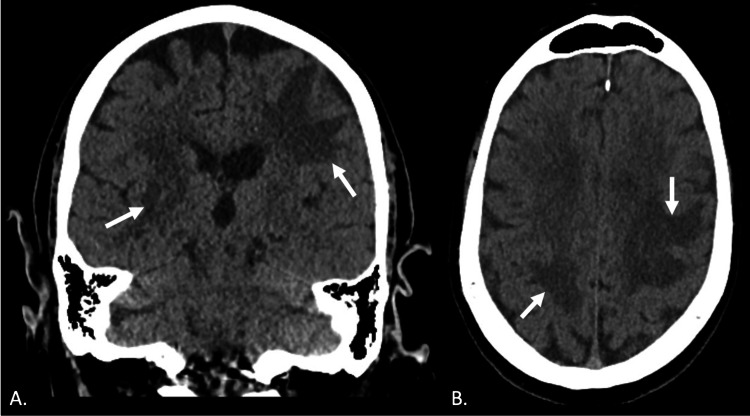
CT of the head without contrast: coronal (A) and horizontal (B) sections with arrows indicating areas of vasogenic edema
